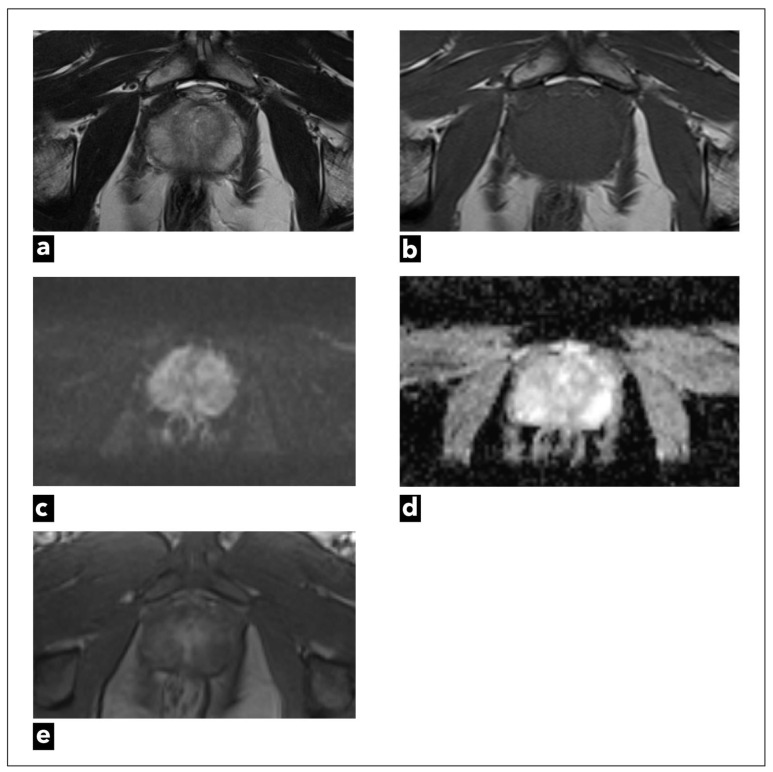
A 47-year-old male patient underwent mpMRI due to a slightly elevated serum PSA level. There were no suspicious findings. Figures present original examples of standard anatomical and functional sequences, respectively: (a) T2W and (b) T1W; (c) DWI; (d) ADC map; and (e) DCE.
